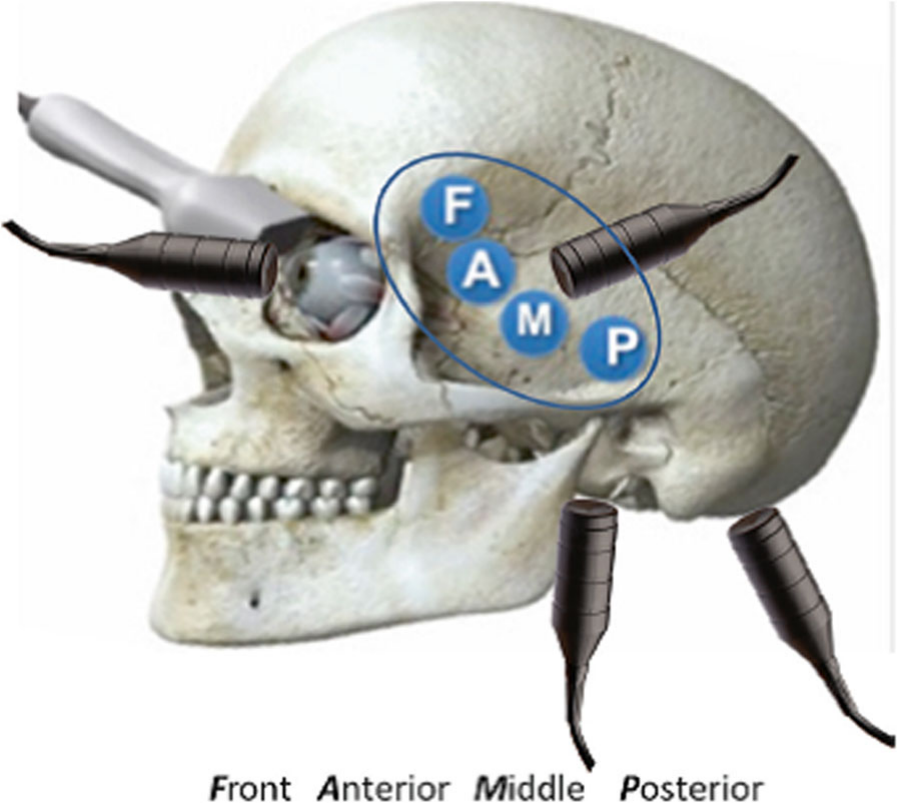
Main approaches to transcranial ultrasonography
